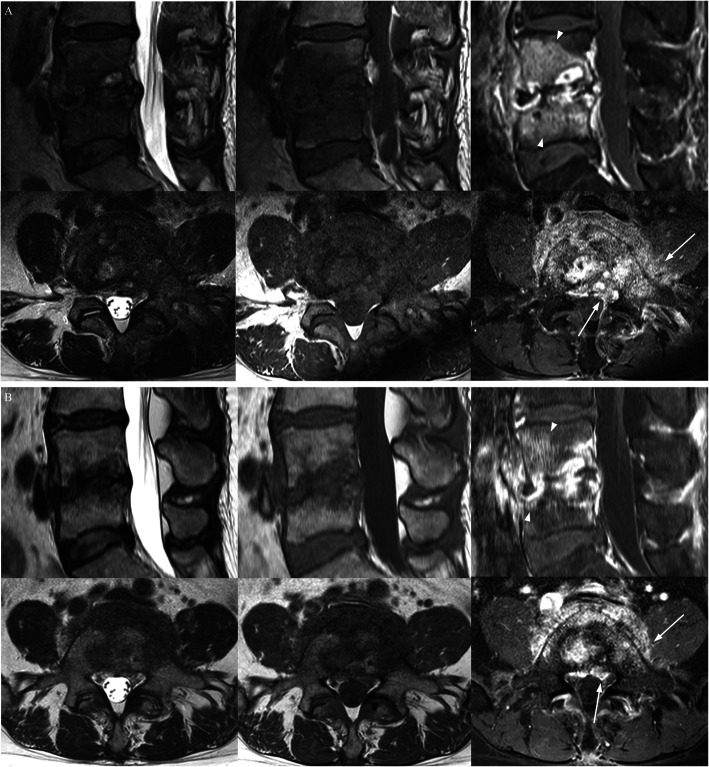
A patient with spondylodiscitis at L4–5 (culture (−), F/U interval 55 days). T2-weighted (left), T1-weighted (middle), and contrast-enhanced fat suppressed T1-weighted images (right) of the spine. Comparing contrast-enhanced fat suppressed T1-weighted sagittal (upper) and axial (lower) images of baseline (a) and follow-up (b), osteomyelitis (arrow heads) and soft tissue inflammation (arrows) were improved, while disc height worsened. Both CRP and ESR were resolved at follow-up
